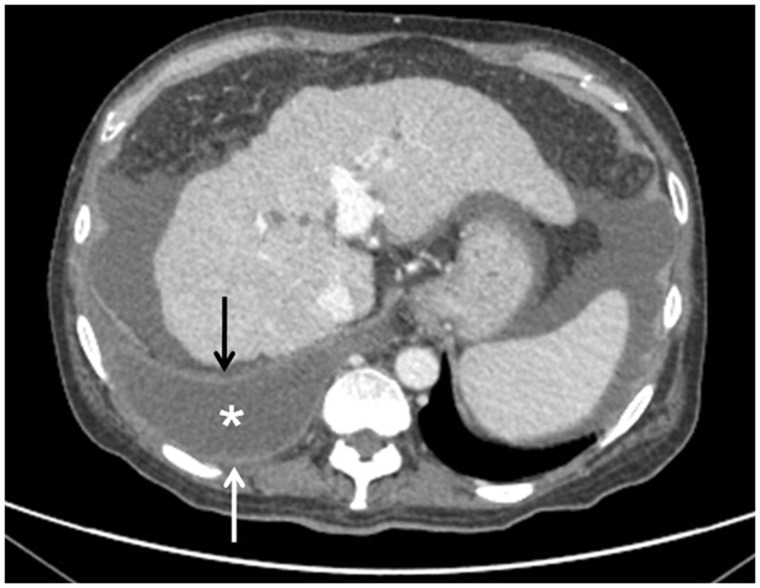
Subacute bacterial empyema. Axial contrast-enhanced CT scan in a known case of cirrhosis showing empyema (asterisk) in right pleural cavity with enhancement of pleura (arrows). Ascites and changes of cirrhosis in liver are also noted.
